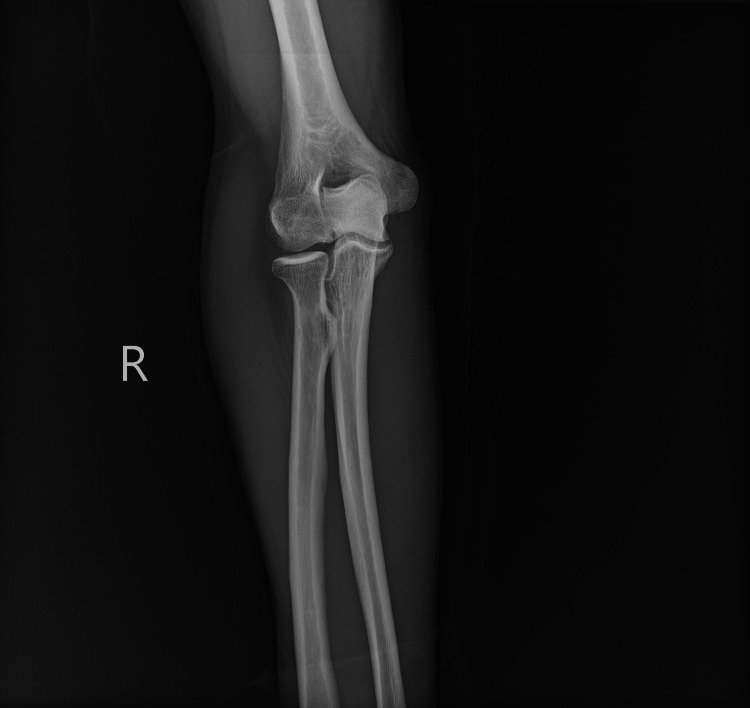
Posteroanterior View Right Forearm with Elbow Joint X-ray
